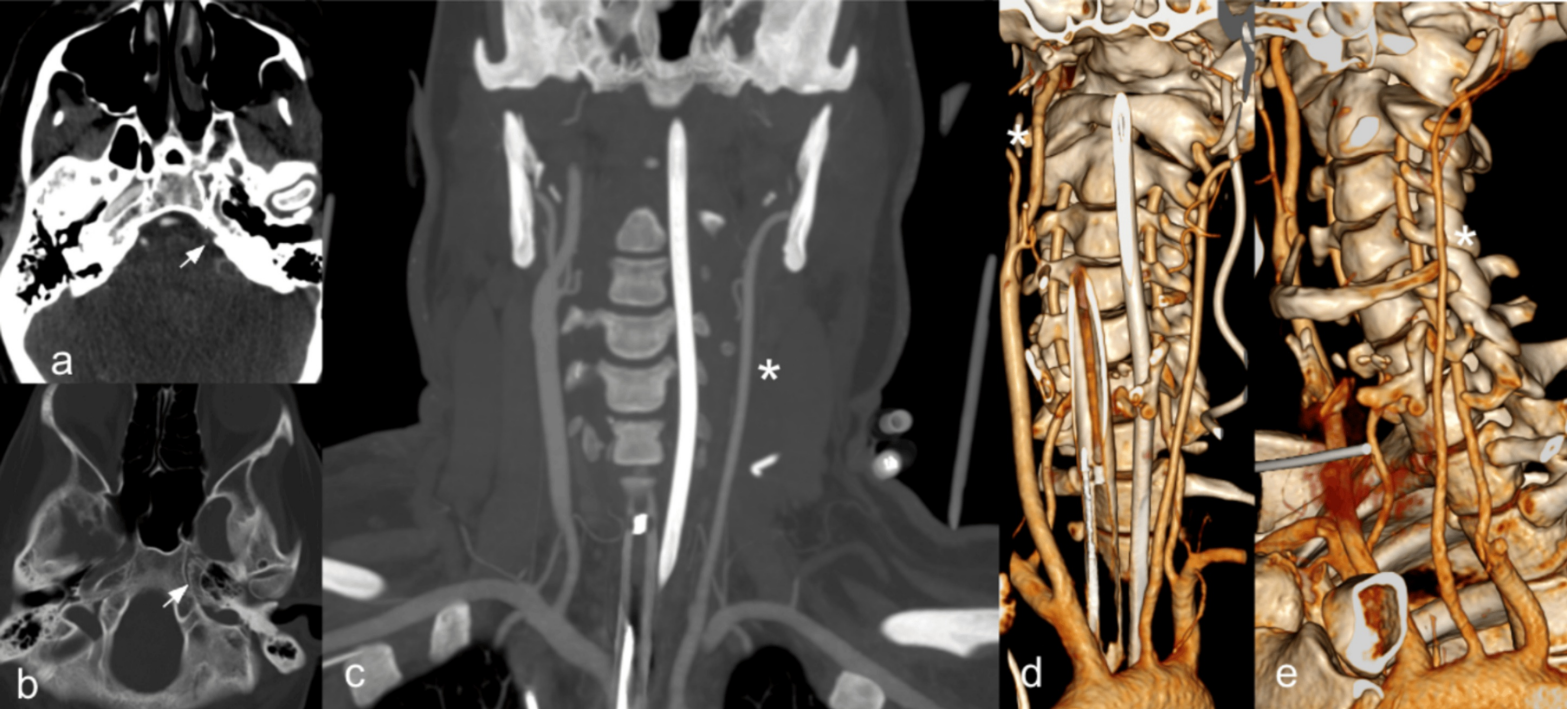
CTA of the head and neck Images in the axial (a, b) and coronal (c) plane, as well as coronal 3D reconstructions (d, e) demonstrating congenital absence of the left ICA and left carotid canal (white arrow). The left CCA can be seen originating from the aortic arch and giving rise to the left external carotid artery (*). ICA: internal carotid artery; CCA: common carotid artery
